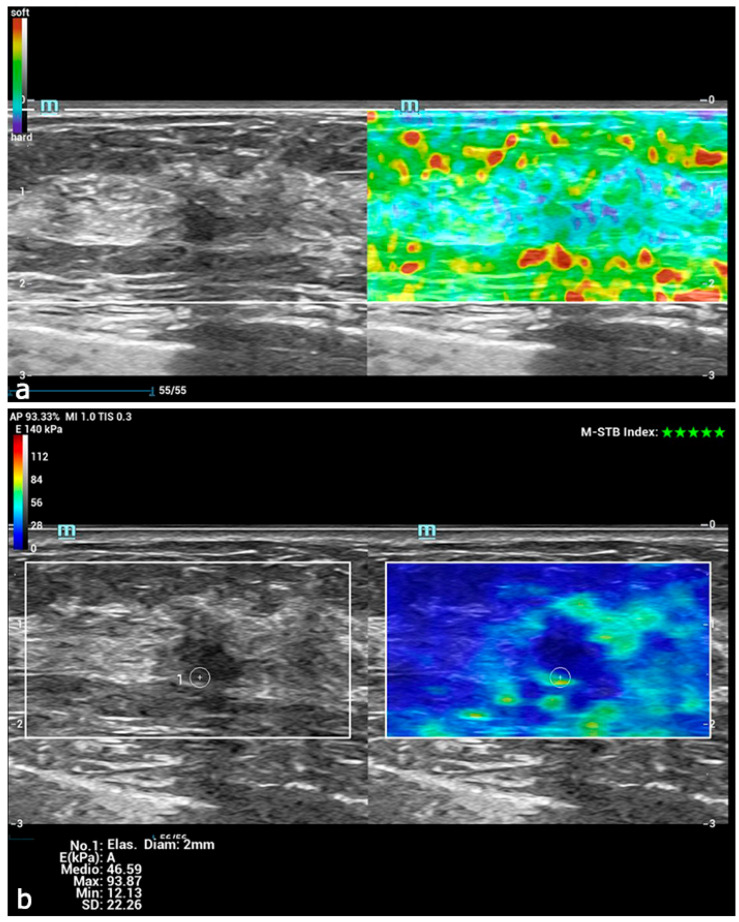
Strain Elastography (SE) (a) and Shear Wave Elastography (SWE) (b) assessments were performed during the same ultrasound examination. SE displayed a green color on the colorimetric map, indicating an intermediate degree of stiffness. SWE measured an average stiffness of 46 kPa in the stiffest part of the lesion, which is a value significantly lower than those reported in the literature for differentiating benign and malignant lesions [20]. The available literature on the ultrasound imaging of breast neuroendocrine tumor (NET) metastases is limited and does not provide reliable criteria with which to distinguish metastatic lesions from primary breast tumors. However, reported cases describe a variety of imaging features. Most lesions appeared as hypoechoic, irregular masses, while others were isoechoic to surrounding fat with a thin echogenic halo. In some instances, lesions exhibited increased acoustic transmission, suggesting possible complex cysts. None of the reported cases demonstrated posterior acoustic shadowing. Color Doppler evaluation frequently revealed marked vascularity, though some lesions showed no detectable flow on power Doppler. Rigid appearance at Strain Elastography has also been reported [14,15].
